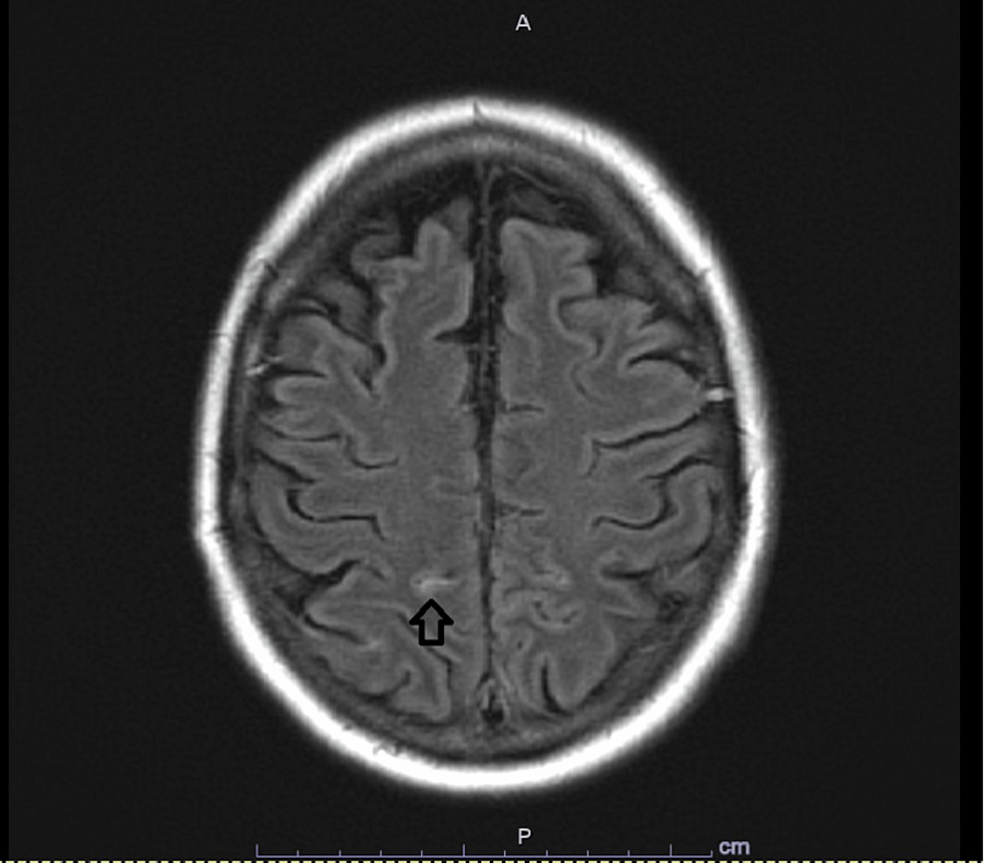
MRI Brain MRI fluid attenuation inversion recovery (FLAIR) brain showing evidence of subarachnoid hemorrhage (arrow)
